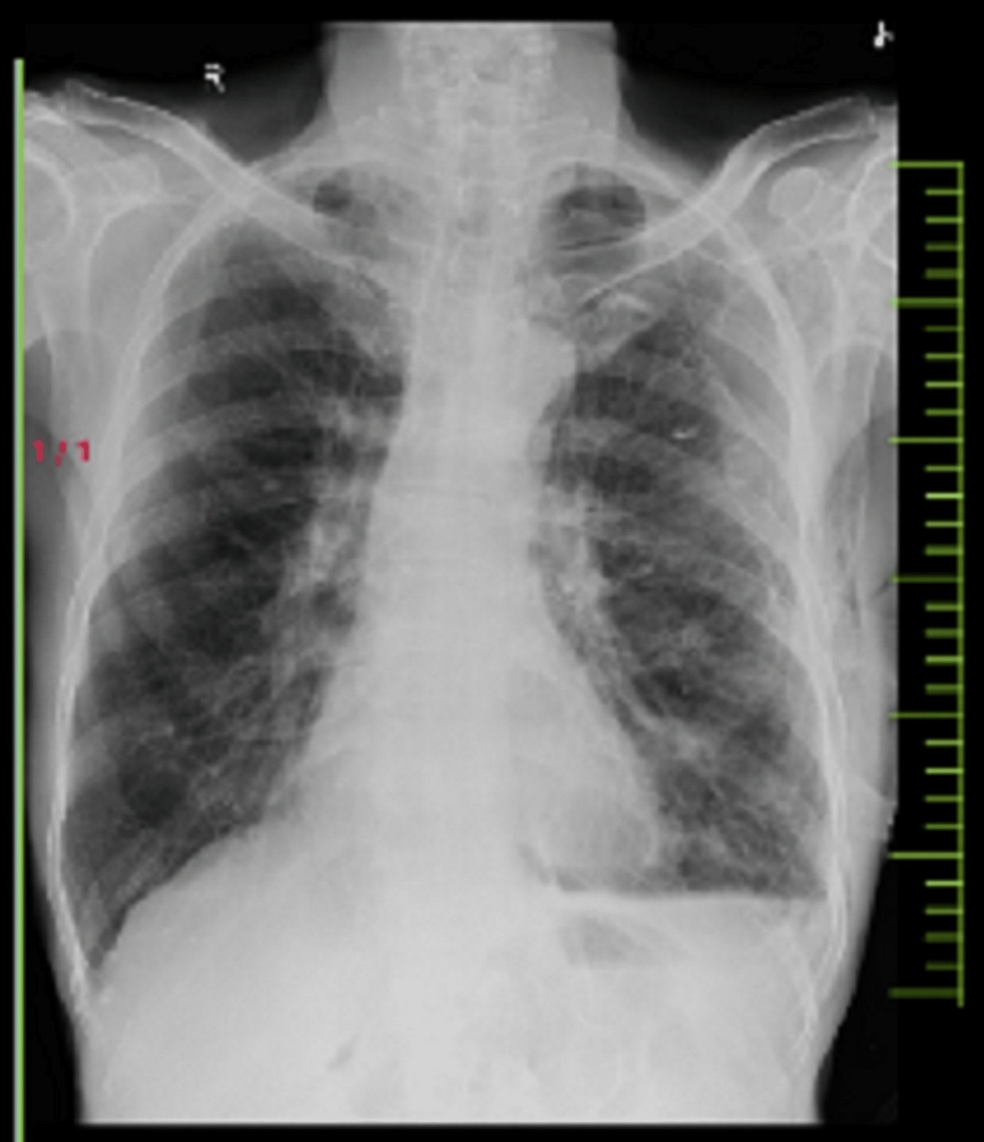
Posterior-anterior view of chest X-ray showing tracheal deviation and flattening of left hemidiaphragm
